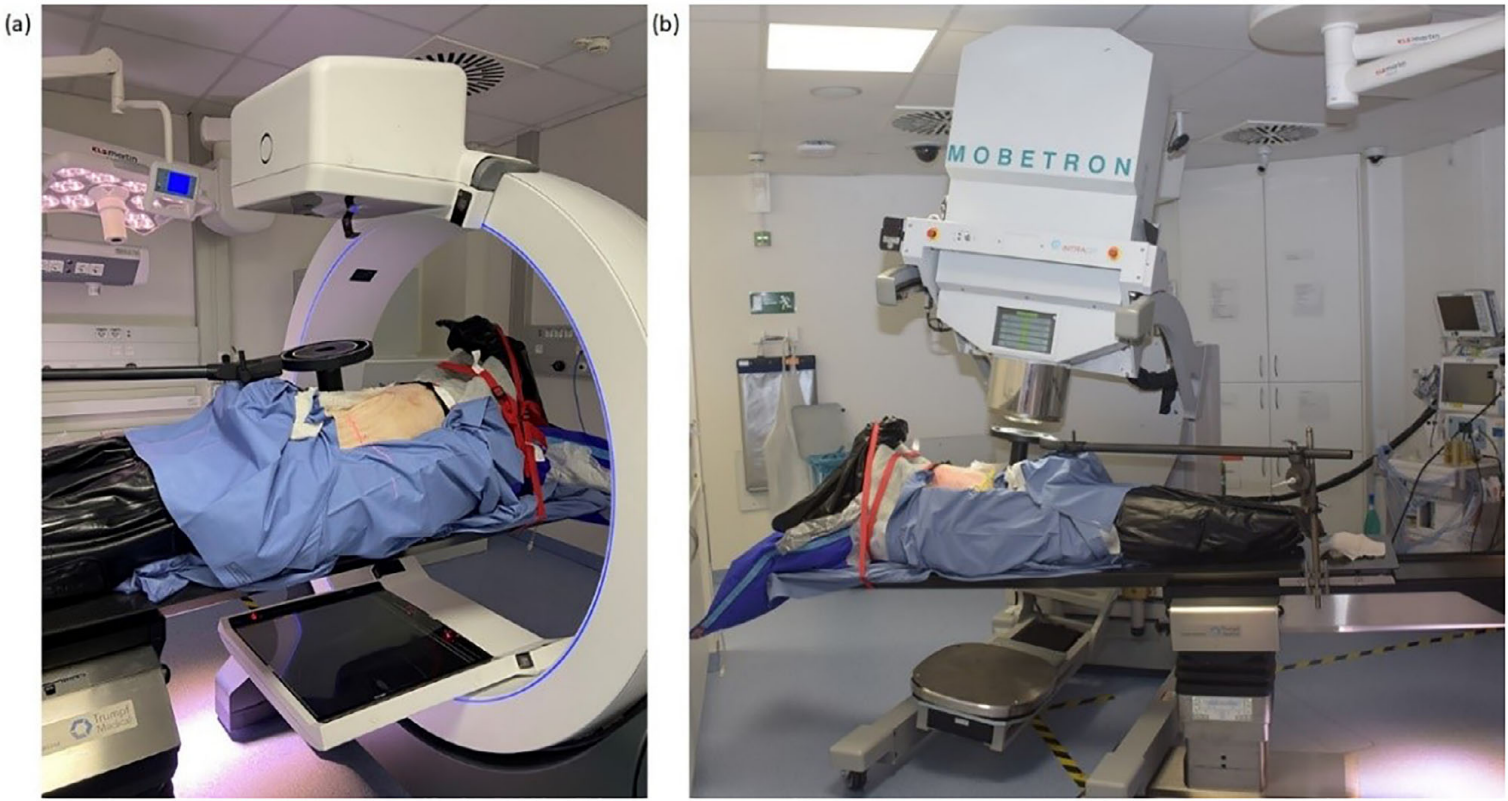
(a) ImR CBCT intraoperative acquisition and (b) treatment delivery with Mobetron LINAC showing the air gap to the clinical applicator.
